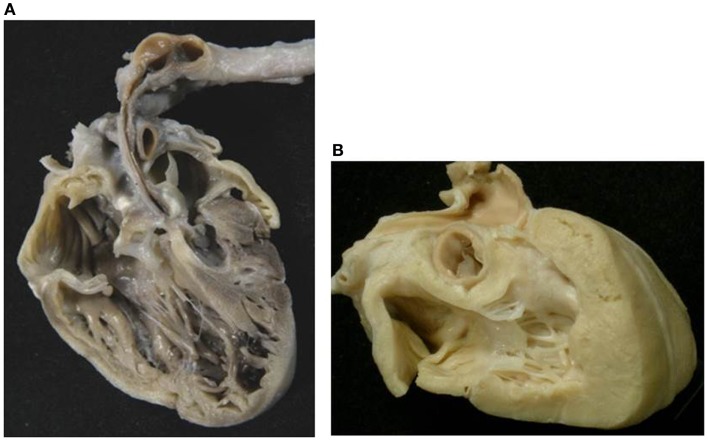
Single outlet ventriculo-arterial connections with functionally univentricular heart. (A) Aortic atresia with intact ventricular septum (single pulmonary outlet). Four chamber echocardiographic cut of the heart showing the severe hypoplasia of the left cardiac chambers. Note the tiny inlet portion of the left ventricle. This heart is only suitable for Norwood univentricular repair. (B) Pulmonary atresia with intact ventricular septum (single aortic outlet) with hypoplastic right ventricle. The size of the right ventricle is such that this heart may be suitable for one and half ventricle repair.
